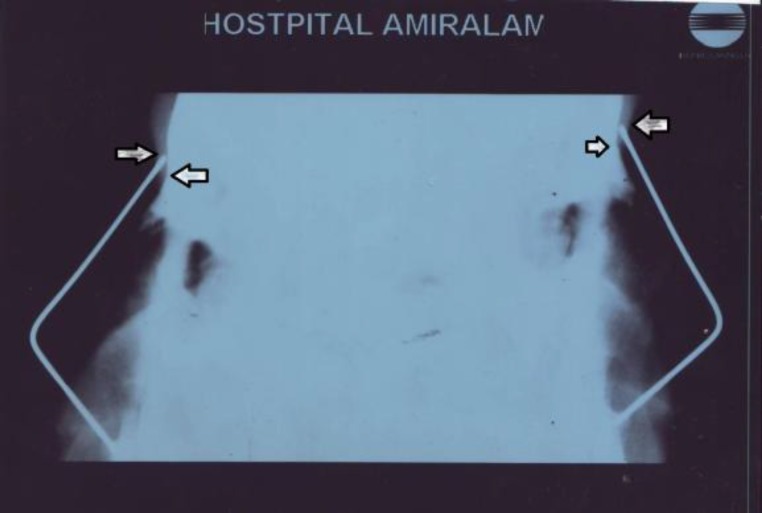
Lateral nasal X-ray of the same patient. The cephalic portion of the wire is
